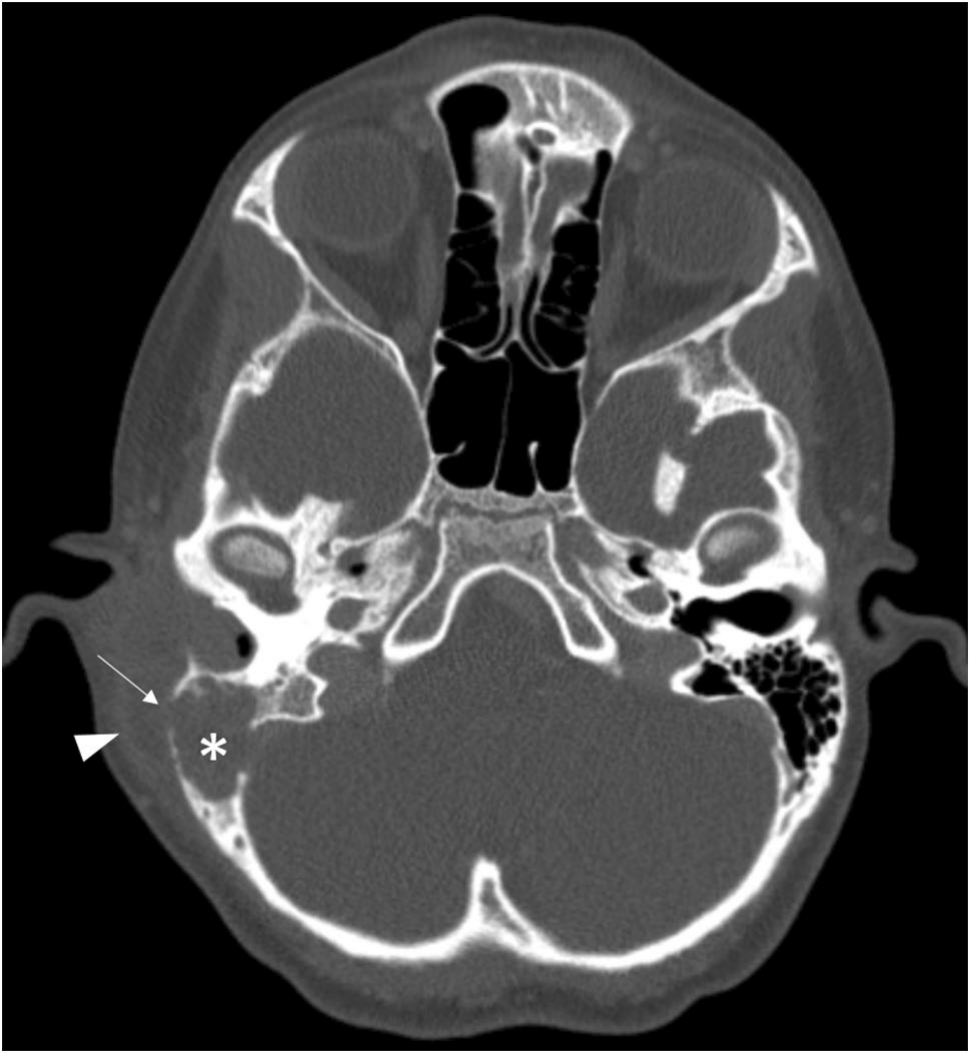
Axial computed tomography image (bone window) without contrast agent administration in a 13-year-old boy with right mastoiditis (*asterisk*) and osseous destruction (*arrow*) and extraosseous periauricular abscess (*arrrowhead*)
